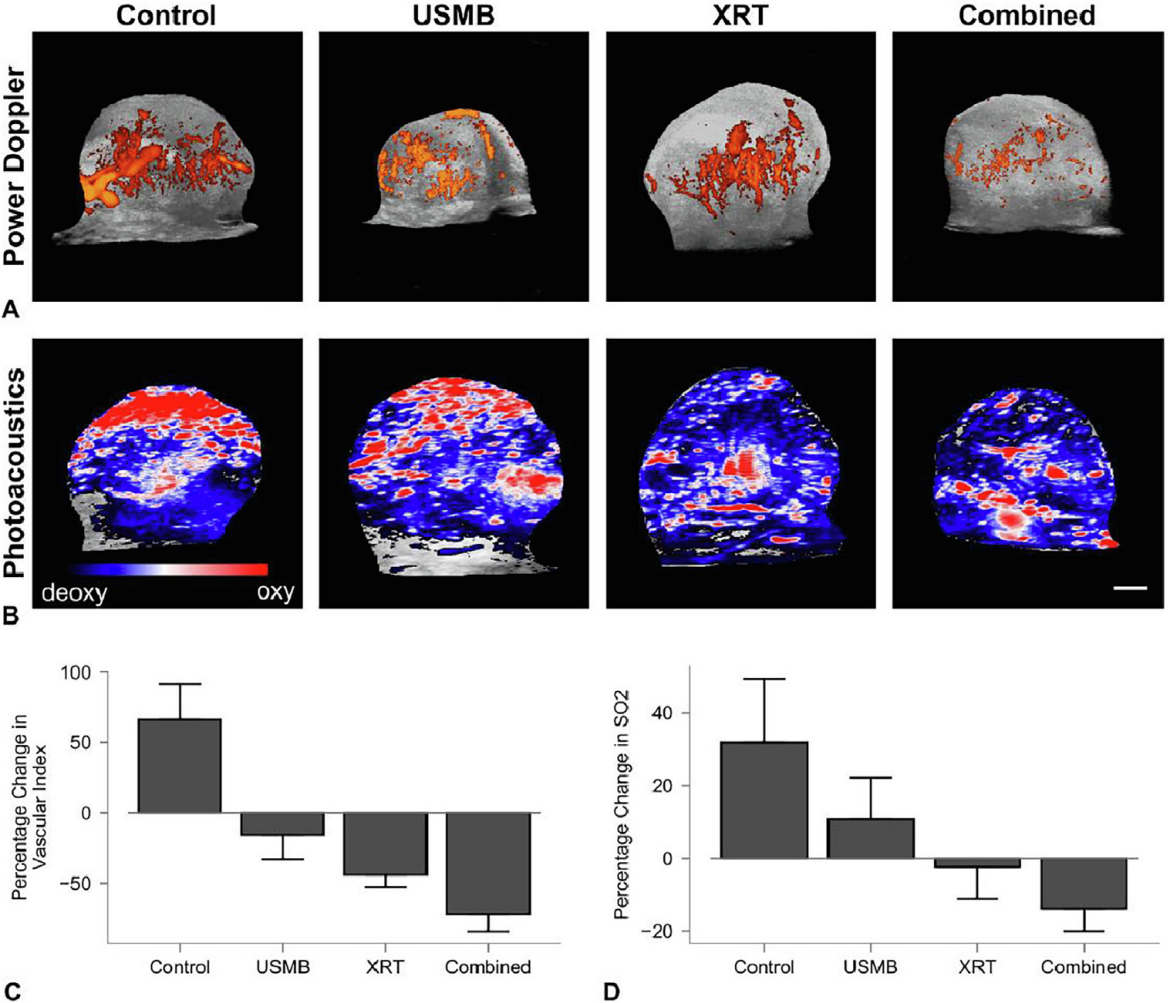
(A) Volumetric 3D power Doppler and (B) photoacoustic post-treatment images of PC3 xenograft tumors *in vivo* 24 h after treatments. For power Doppler imaging, colored overlays indicate 0–40 dB. For photoacoustic imaging, the blue and red color bar represents a relative ratio of oxy- and deoxyhemoglobin levels, respectively. The scale bar represents 4 mm. Treatment conditions included untreated controls, 1% (v/v) microbubble and focused ultrasound exposure (USMB), (XRT, 8 Gy), and combined treatments (USMB + XRT). (C) Percent change in vascular index depicting diminishment in blood flow following combined treatment compared to control groups. (D) Percent change in oxygen saturation showing oxygen saturation reduction in combined treated group comparing control groups. Error bars represent the standard error of the mean (SEM) [80].
